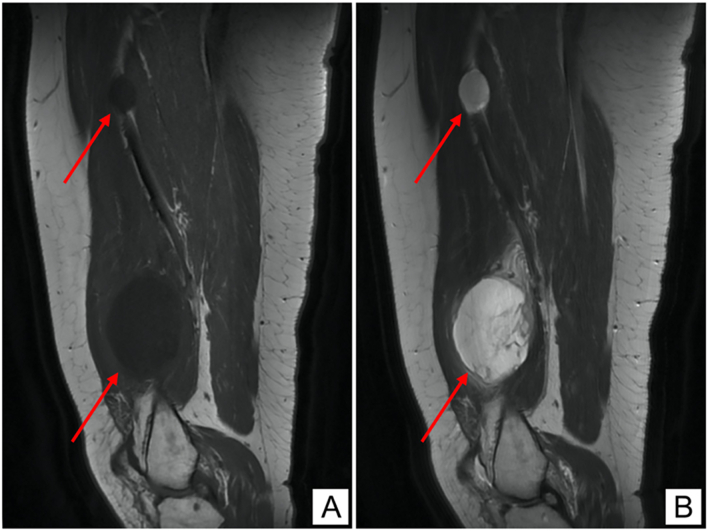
(A) Sagittal MRI section showing low signal intensity on T1W imaging in two soft-tissue lesions. (B) Sagittal MRI section showing high signal intensity on T2W imaging in two soft tissue lesions.
